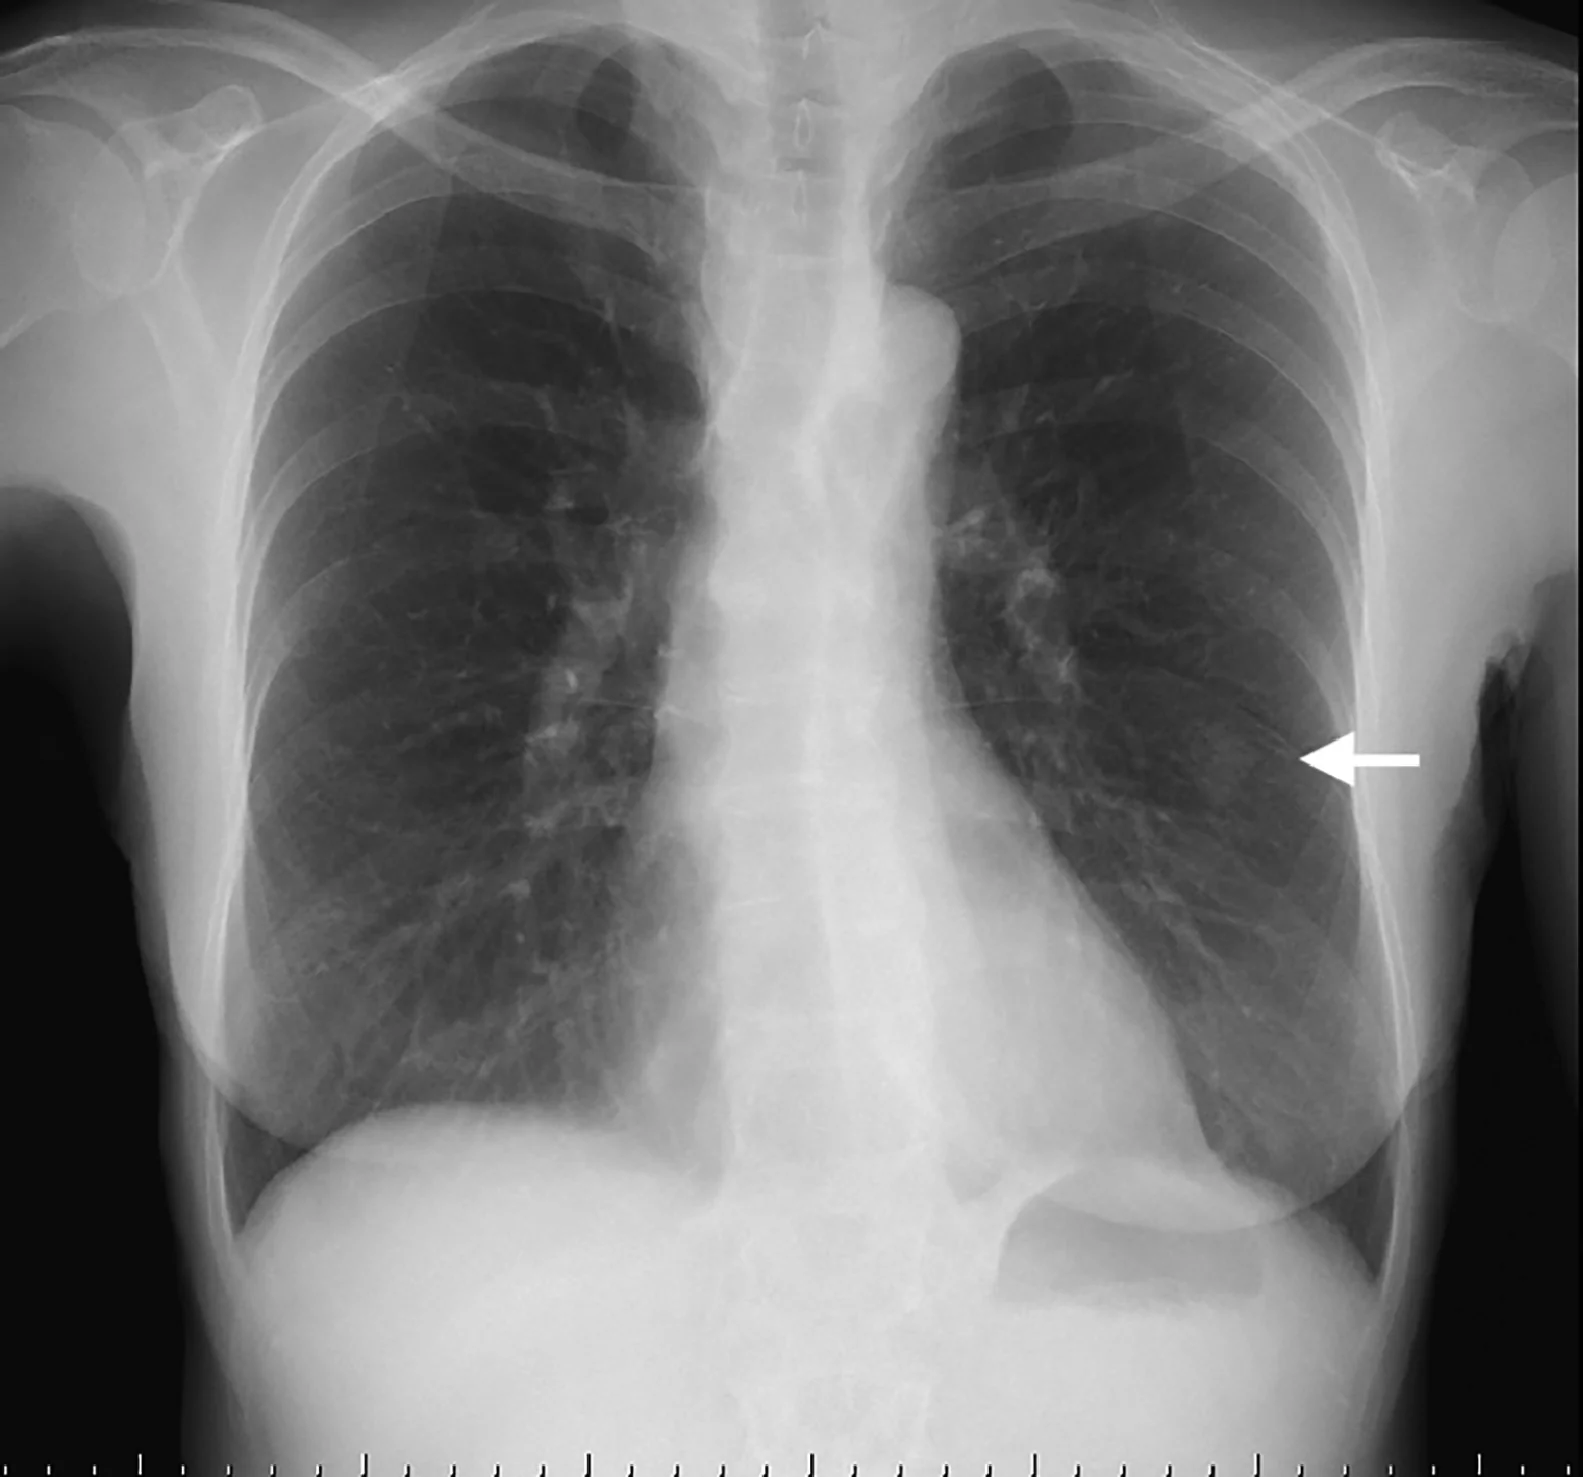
Chest radiograph reveals a nodular opacity in the left lower lung field (arrow), without obvious calcification or cavitation, prompting further evaluation with computed tomography.
